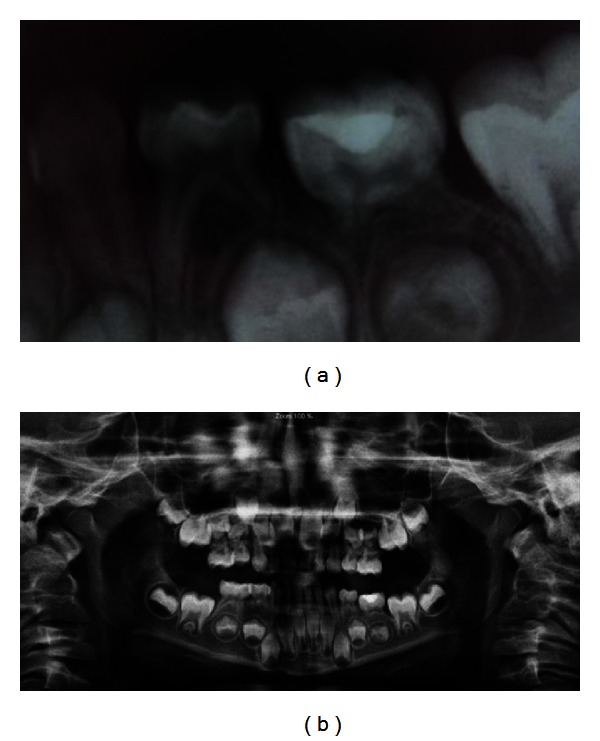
(a) Ten-month periapical view. (b) Ten-month panoramic view.
